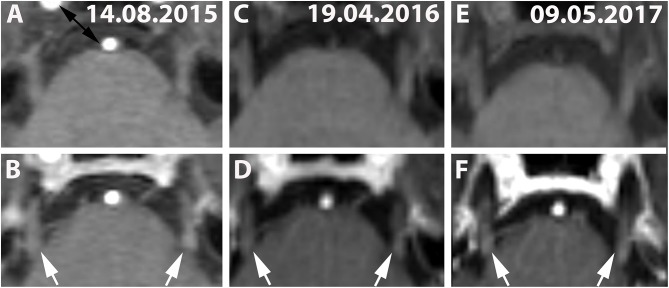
(A,C,E) (Patient No. 8): Pre-contrast and corresponding post contrast T1-weighted axial images (B,D,F) reveal long-lasting bilateral (left-dominated) trigeminal enhancement (white arrows) of >20 months on three follow-up MRIs (A,B: 14.08.2015; C,D: 19.04.2016; E,F: 09.05.2017). No brainstem lesion was detected in this case on T2-weighted images (not shown).
